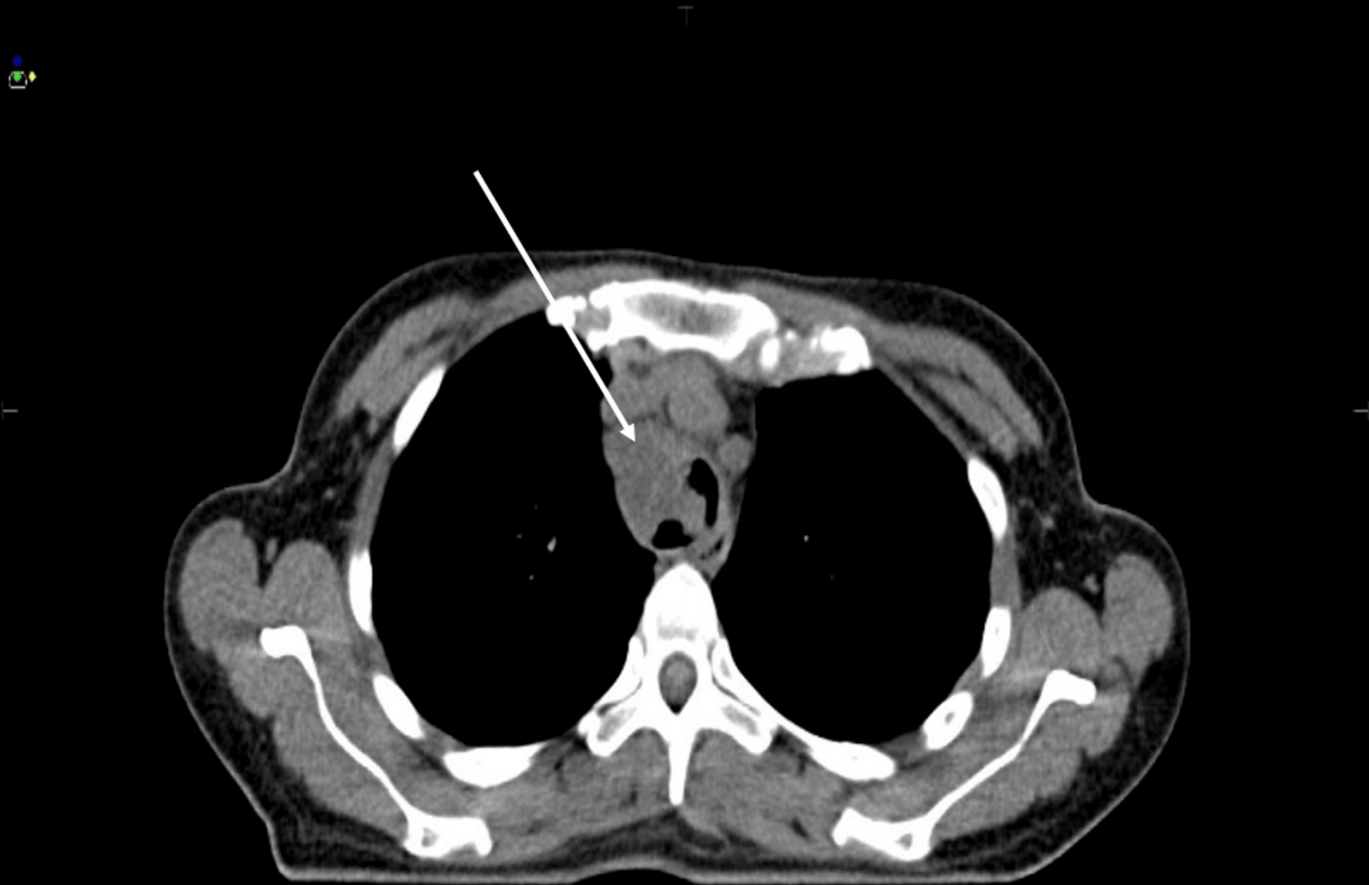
Axial CT image showing the lower extension of the tracheal lesion. CT, computed tomography.
